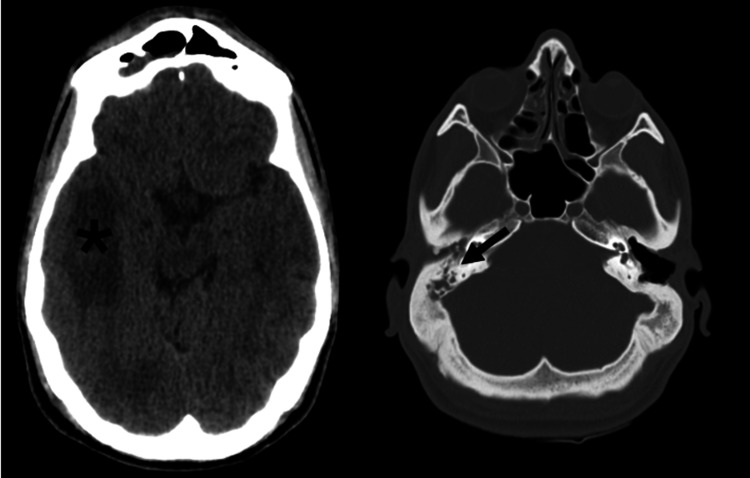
Brain CT with bone erosion (black arrow) and temporal cerebritis (*) with a possible abscess and edema. CT: computed tomography
